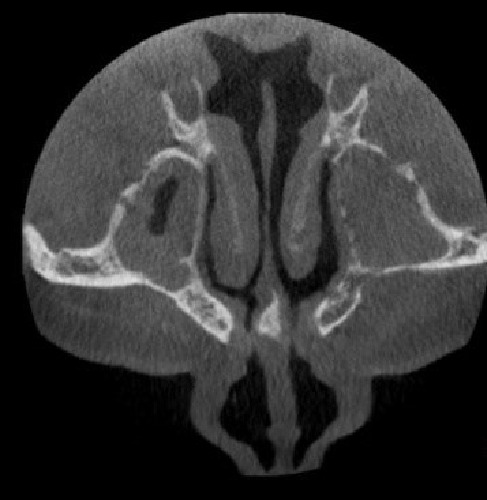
bone destruction and haziness in maxillary sinus shown in axial cone beam computed tomography (CBCT) imaging
